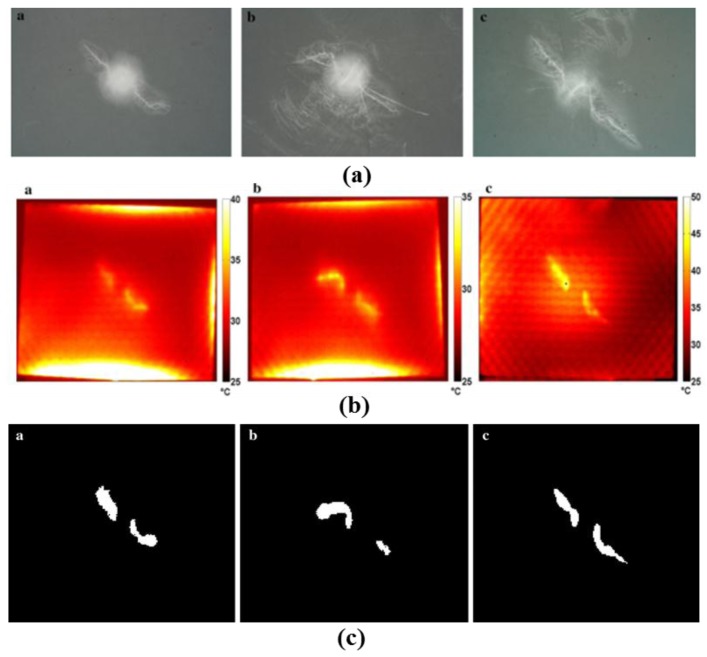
X-ray images of three samples (a), T_max_ maps during all acquisition sequence (b), and binary images obtained by T_max_ maps (c) [75]. Reprinted/reproduced with permission from Springer.
